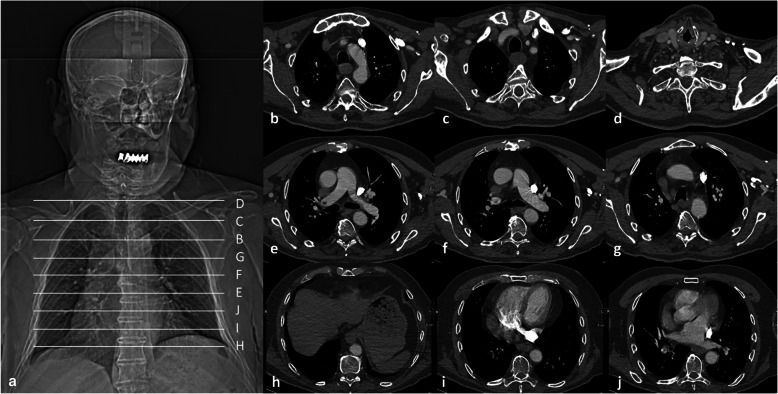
Examples of anatomical landmarks on axial CT angiography source images. a Shows a topogram of a CT angiography with reference lines corresponding to anatomical landmarks depicted in (b-j). Axial CT angiography images at the level of the aortic arch (b), supra-aortic vessels (c), cervical arteries (d), main pulmonary artery (e), carina (f) and ascending aorta (above the carina (g). The diaphragm and abdominal organs are shown in (h), the ventricles and left atrium in (i) and (j). Note: Depending on the level of the most caudal axial image the following lung segments are usually, at least partially, covered by CT angiography: B = right: 1–3, left: 1 + 2 and 3; C = right: 1 and 2, left: 1 + 2; D = no lung tissue; E = right: 1–3 and 6, left: 1 + 2, 3, 4 and 6; F = right: 1–3 and 6, left: 1 + 2, 3 and 6; G = right: 1–3, left: 1 + 2, and 3; H = right: 1–10, left: 1–10; I = right: 1–10, left: 1–10; J = right: 1–6, left: 1–6, and 8
